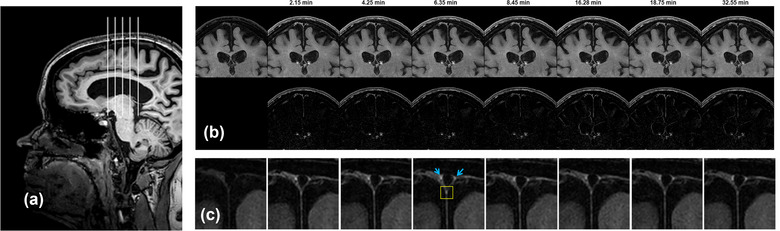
(A) Sagittal T1 images showing the location of the coronal contrast‐enhanced fluid‐attenuated inversion recovery (T2‐FLAIR) images. (B, upper row) Coronal FLAIR images before and at multiple time points (in minutes; [min]) after contrast administration in a 53‐year‐old female patient with relapsing‐remitting MS. (B, lower row) difference images relative to the precontrast scan. (C) Zoomed view showing the region of interest where contrast enhancement in the inferior dural lymphatic vessel (yellow box) was measured. The more superior dural lymphatic vessels are indicated by blue arrows. Note the excellent suppression of dural sinus and cerebrospinal fluid signal throughout the data collection period characteristic of the T2‐FLAIR sequence.
